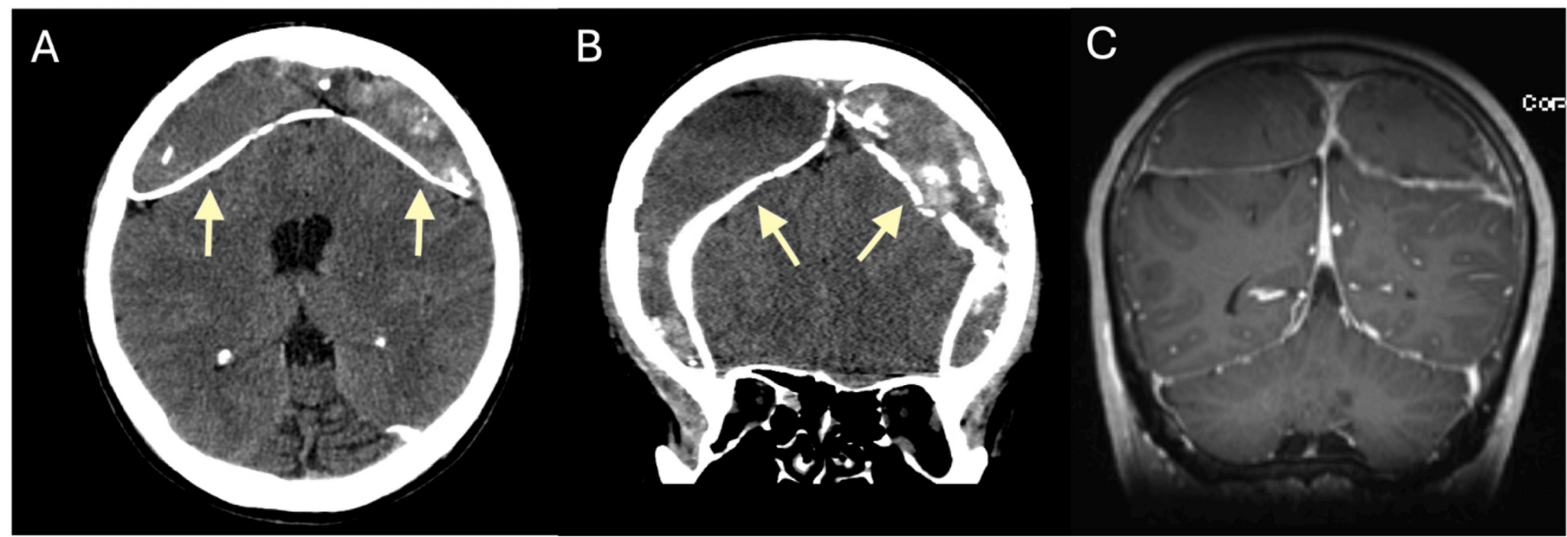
(A) Axial CT with bilateral calcifications (arrows). (B) Coronal CT revealing sulcal effacement and mass effect (arrows). (C) Coronal MRI with contrast with no enhancement of the collections.
